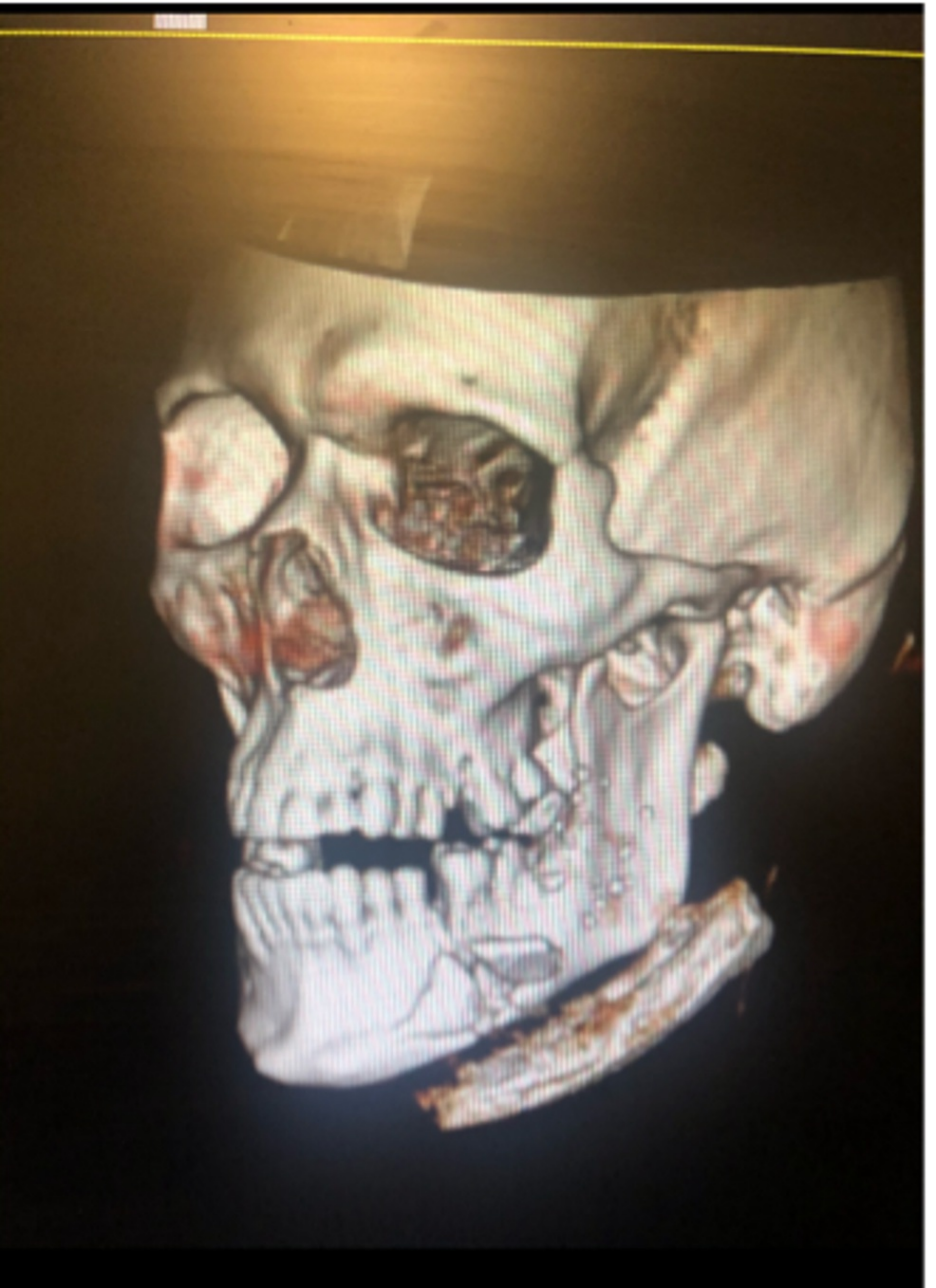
Maxillofacial CT scan demonstrating left mandible fracture.
